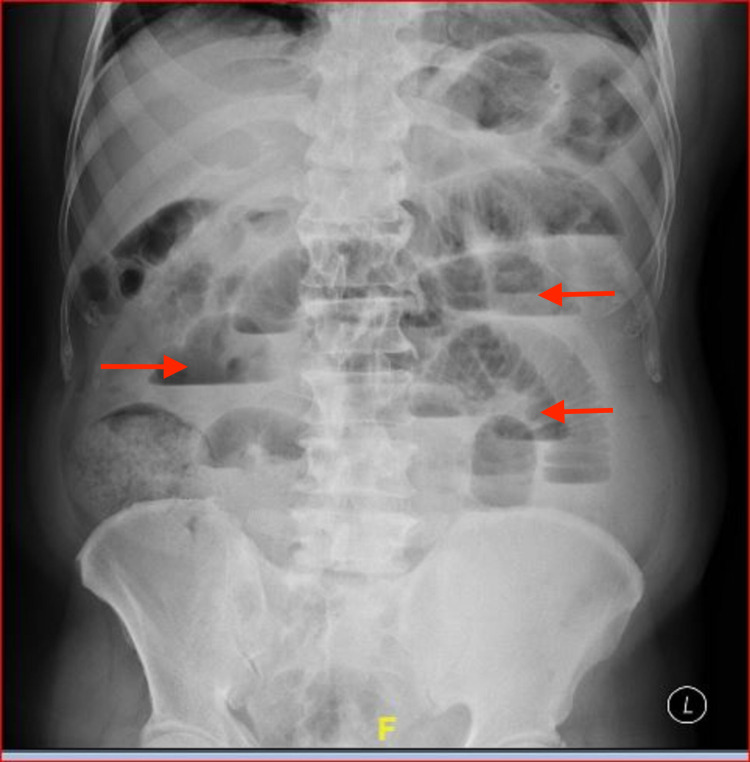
Plain radiograph of the abdomen - erect view showing multiple dilated small bowel loops with air-fluid levels (arrows).
